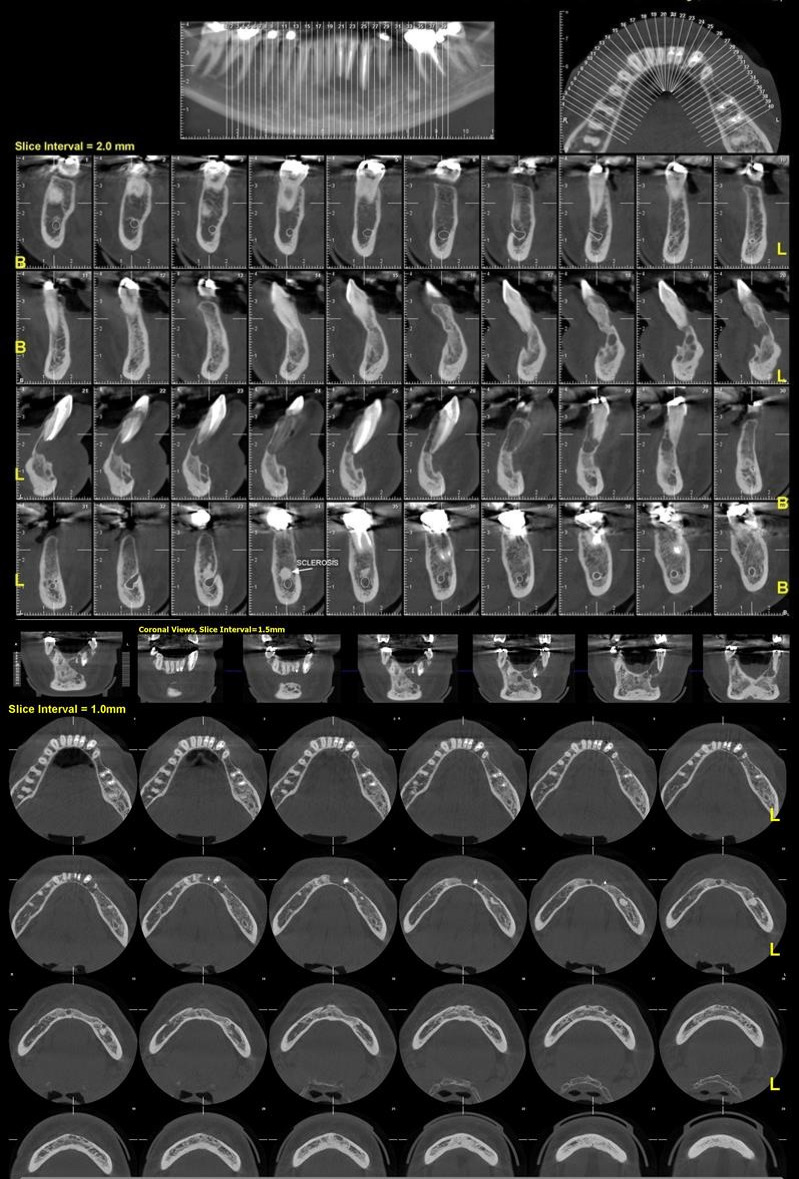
One‐year postoperative follow‐up cone beam computed tomography (CBCT) scan revealing no traces of recurrence
